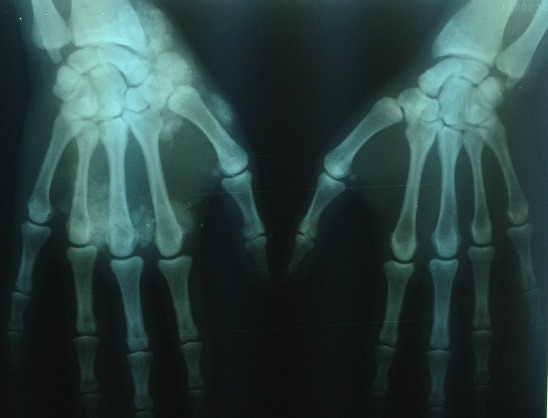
Radiographie standard des deux mains objectivant une calcinose pseudotumorale de la main droite avant par thyroïdectomie
